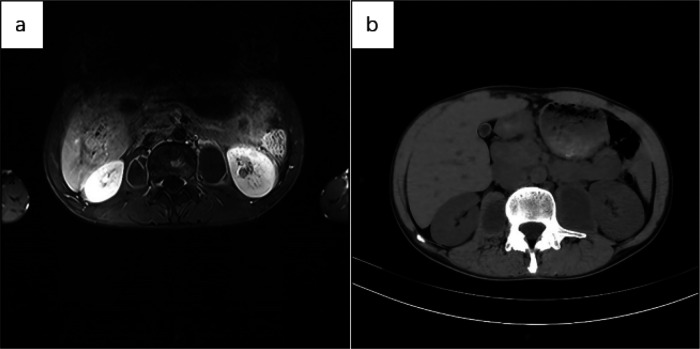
(a) axial MRI of the upper abdomen (T1WI with lipid suppression) showed a patchy hyperintense signal in the lumbar vertebral body and bilateral paravertebral capsules. The cystic walls were smooth, with uniform thickness, and the contents within the capsule exhibited a consistent low signal. Given the medical history, spinal TB with a paravertebral cold abscess was considered. The left kidney showed a small, non-enhancing low-signal area, suggestive of a cystic lesion. (b) Axial CT of the upper abdomen showed no obvious abnormalities in the morphology, size, or density of both kidneys. Bilateral cystic low-density shadows were seen beside the lumbar vertebral body, with uniform wall thickness and no wall nodules. The sacs exhibited a uniform low-density shadow, and no septation was noted.
